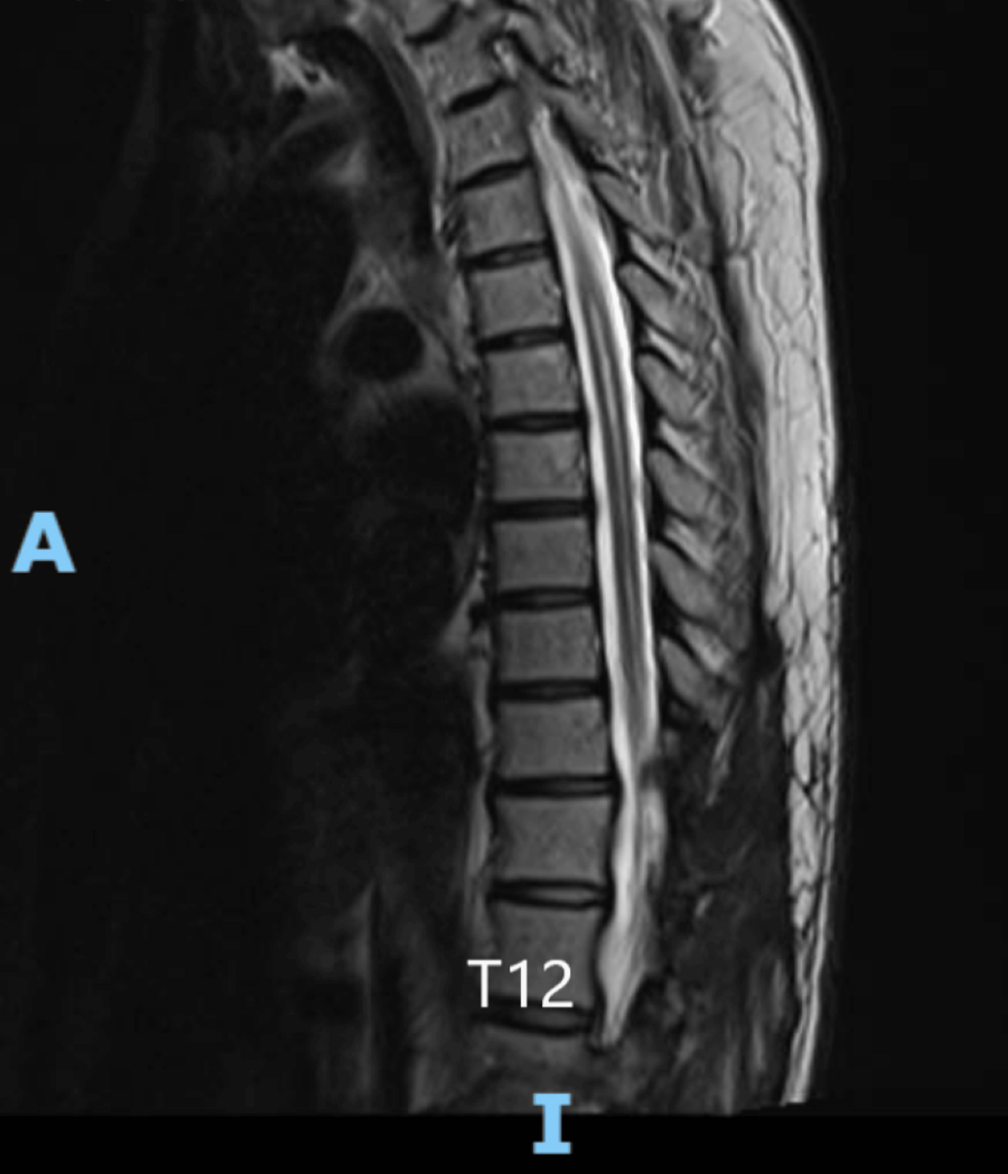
Post-operative sagittal MRI image of intradural lesion at the level of T12 at seven months post operation showing significant improvement of syrinx.
